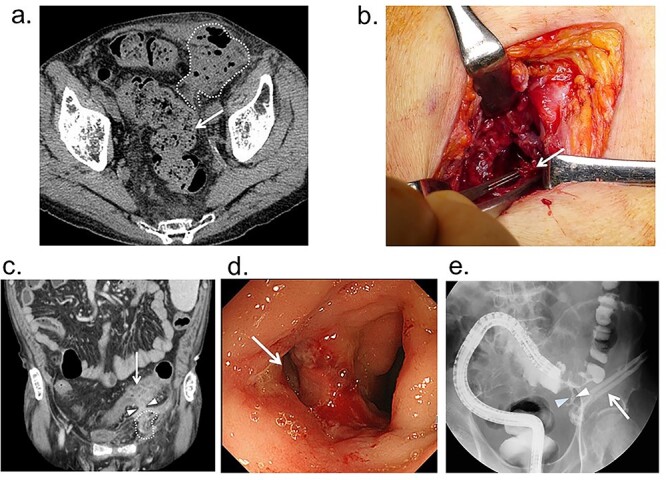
(a) Before drainage abdominal CT findings; abscess is found in the subcutaneous space and preperitoneal cavity of the left inguinal region (dotted-line circle), and it is adjacent to the sigmoid colon (arrow); (b) findings of emergency drainage; a portion of the Kugel patch (arrow) was firmly adherent to the abdominal wall, and the abscess cavity continued further into the mesh, but it was unclear whether it had reached the abdominal cavity; (c) CT findings after drainage: edematous changes in the sigmoid colon and a fistula continuous with the abscess cavity; (d) colonoscopy: ulceration and fistula (arrow) are found in the sigmoid colon; (e) lower gastrointestinal tract radiography findings after drainage: fistula is found (arrowhead) between the sigmoid colon and drainage tube (arrow).
